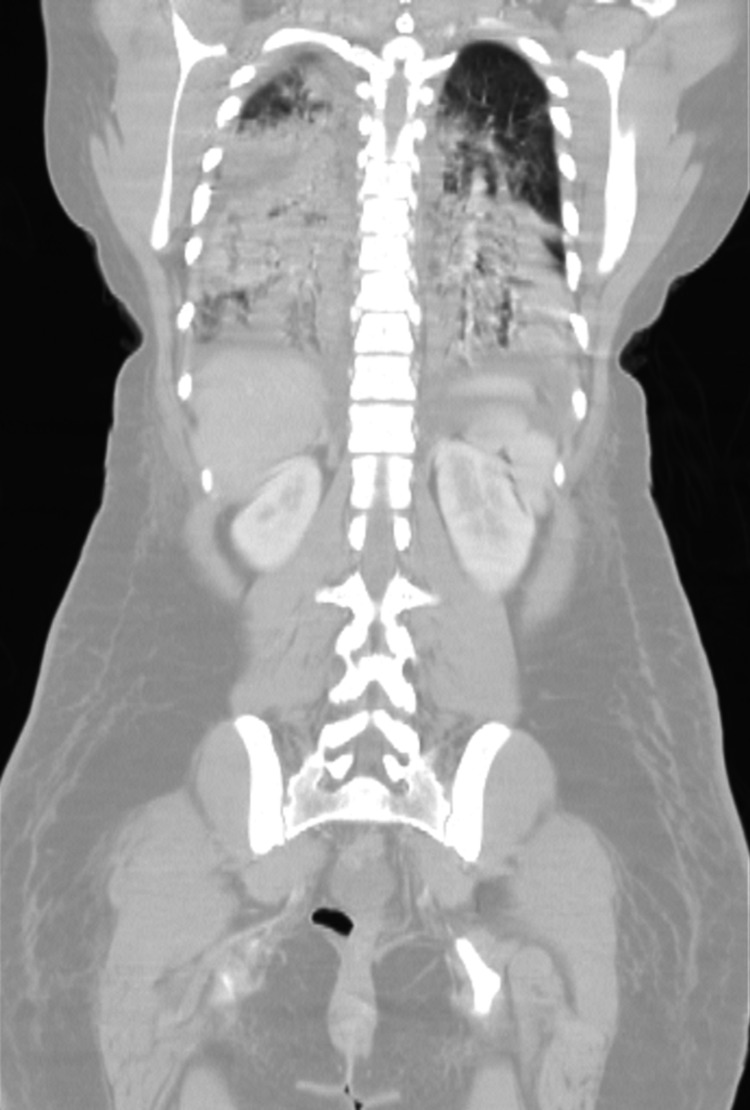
CT chest showing bilateral infiltrates from posterior coronal view
